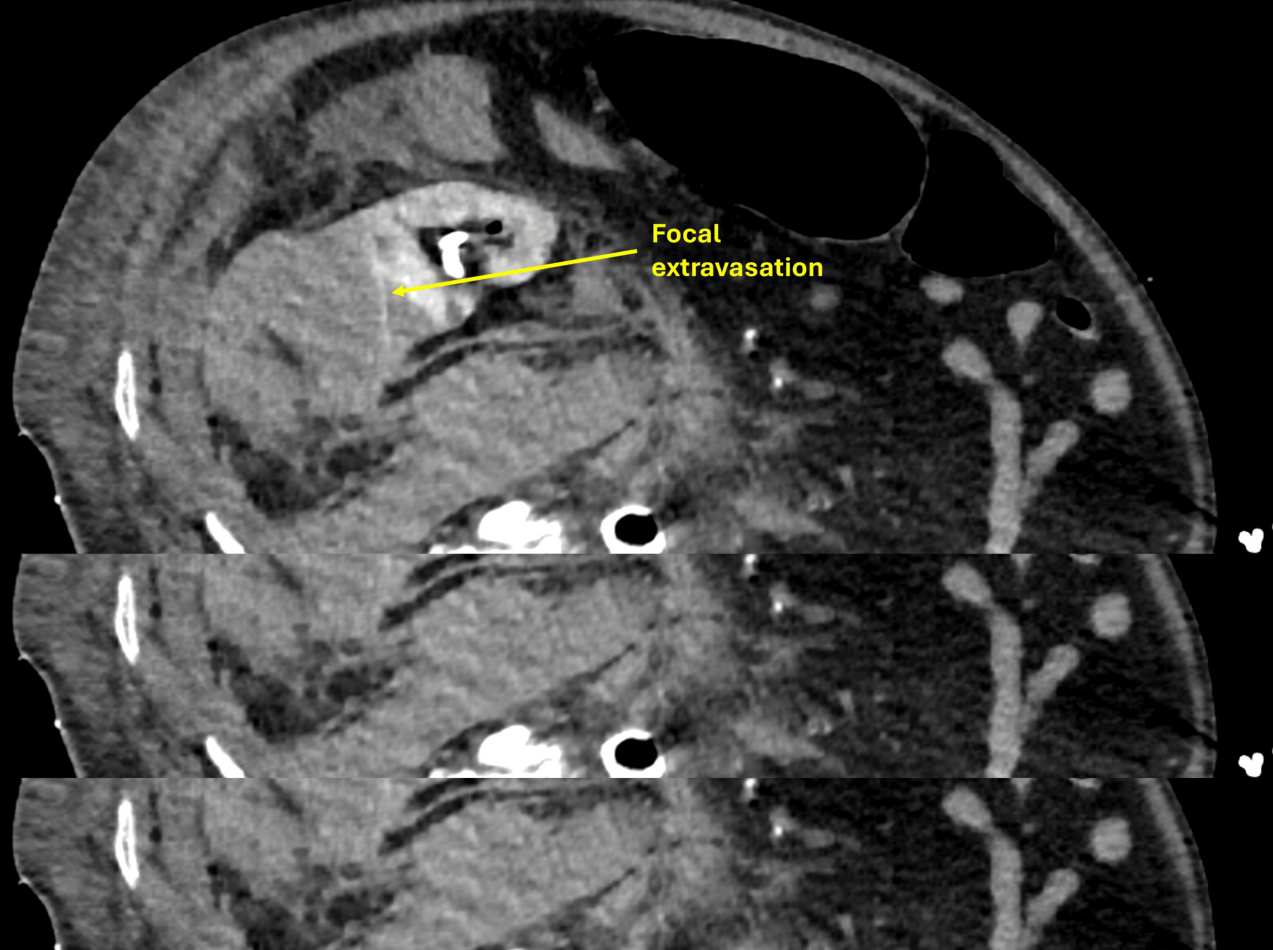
CT image showing right perinephric extravasation at the nephrostomy site.
